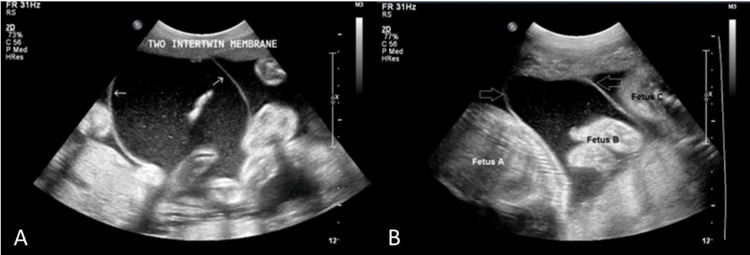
An ultrasonographic image shows two echogenic, intertwined membranes (white arrows in (A) and open white arrows in (B)) among three fetuses.
